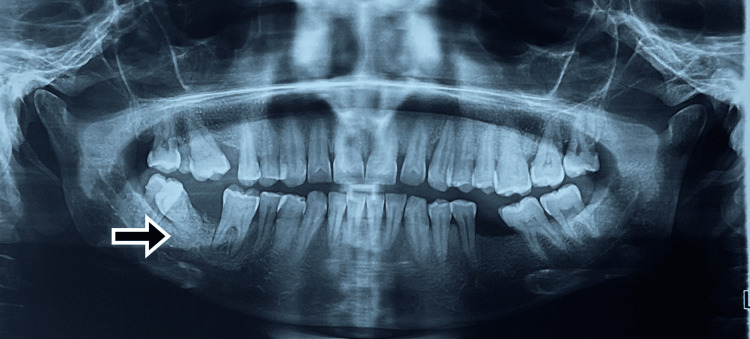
Orthopantomogram showing tilted right second molar
